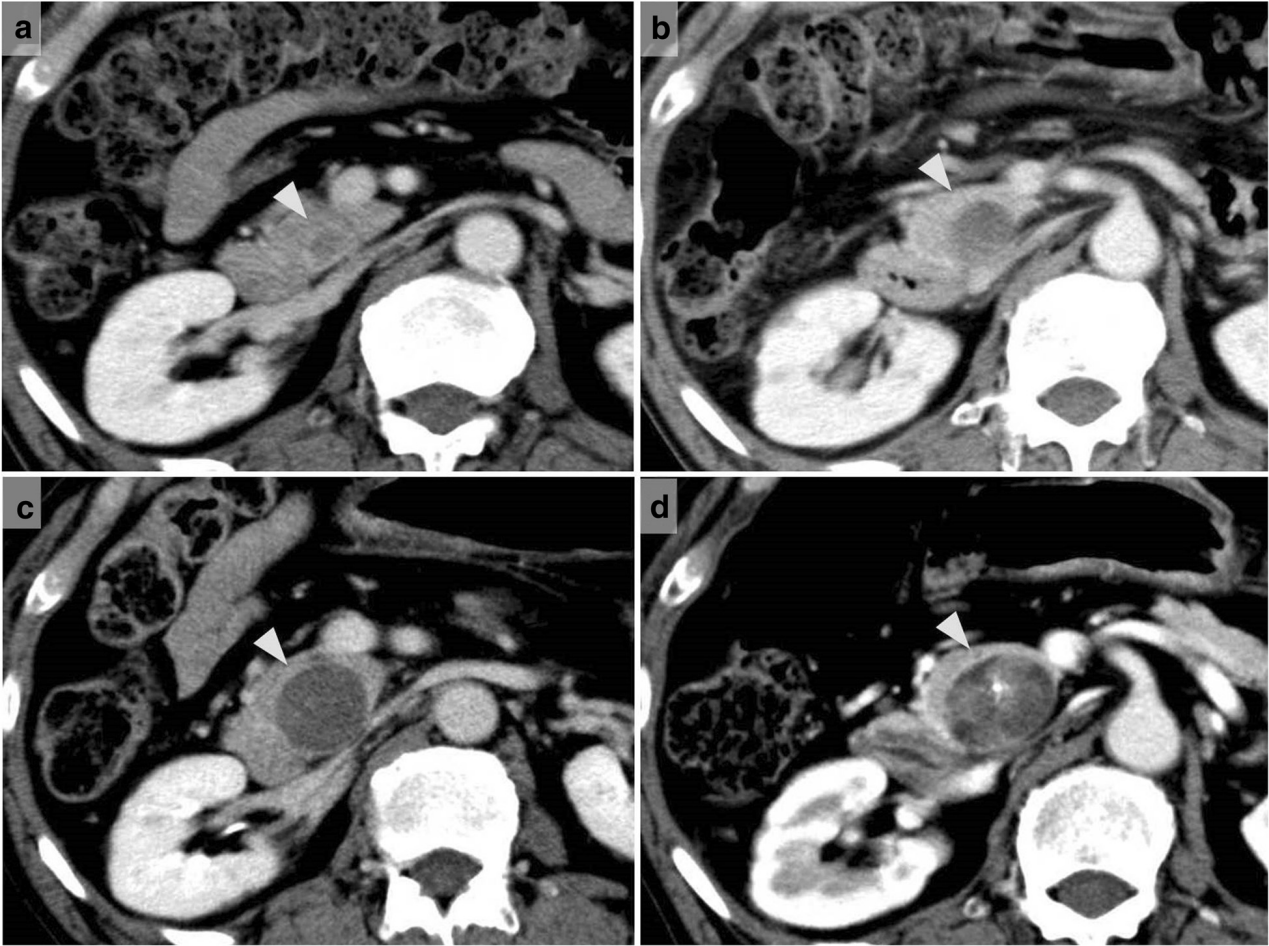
Contrast-enhanced computed tomography revealed the enlargement of the tumor in the intrapancreatic bile duct. CT scans obtained in a October 2005 (2 years and 10 months after hepatectomy) depicted a very low-density mass in the intrapancreatic bile duct. CT scans obtained in b December 2007 (5 years after hepatectomy), c January 2011 (8 years and 1 month after hepatectomy), and d February 2014 (11 years and 2 months after hepatectomy) show gradual dilatation of the intrapancreatic bile duct; the mass had gradually enlarged
